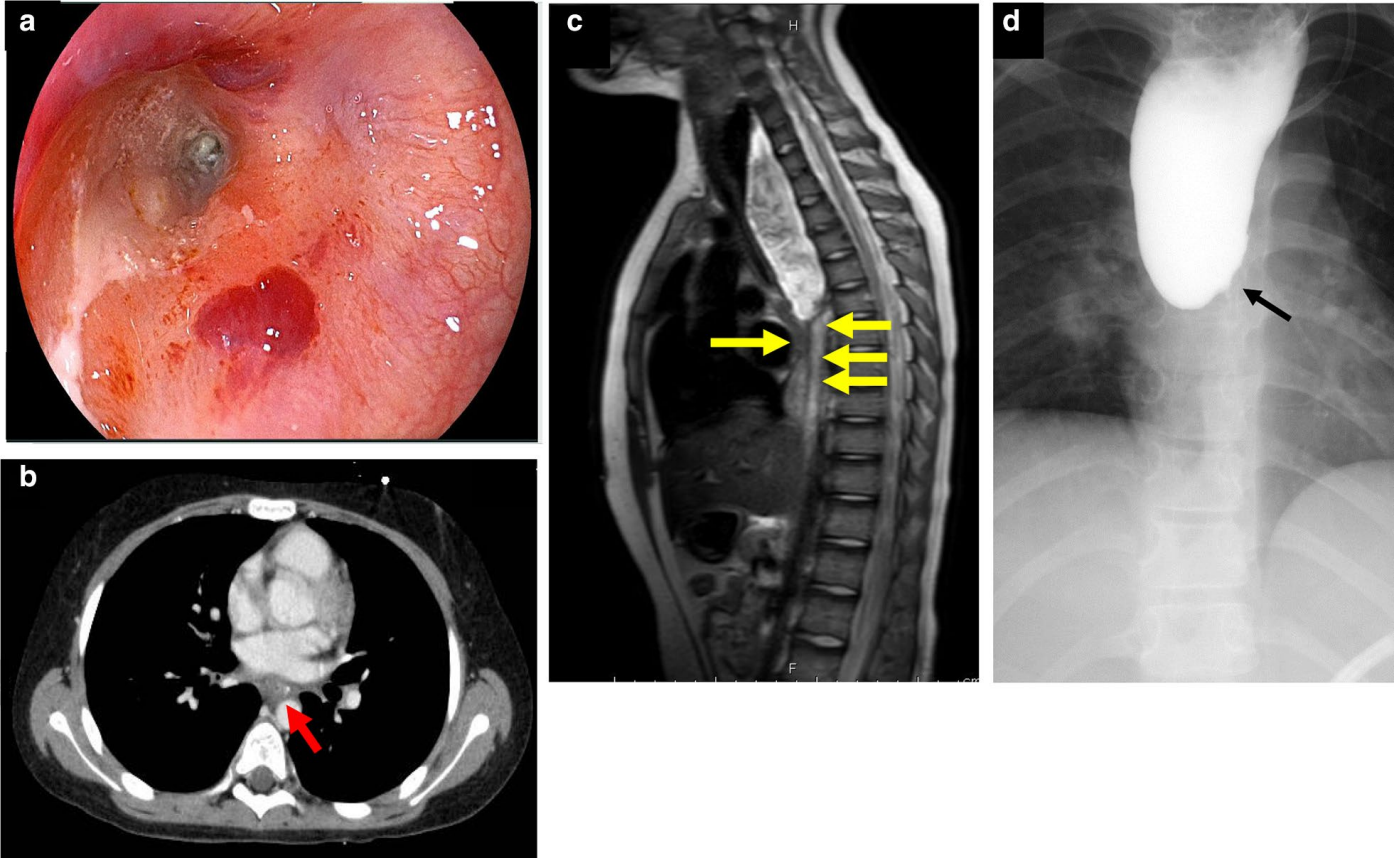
Imaging findings. a Esophagoscopy revealing a severe stricture along with circumferential wall thickening, which made the passage of the scope impossible. b Contrast-enhanced chest computed tomography during chemotherapy revealing reduced lymphadenopathy in the mediastinal lymph nodes (red arrow). c Contrast-enhanced magnetic resonance imaging during chemotherapy revealing the narrowing of the middle thoracic esophagus (yellow arrows). d Barium contrast imaging revealing a complete obstruction (black arrow) in the middle thoracic esophagus with proximal dilation
